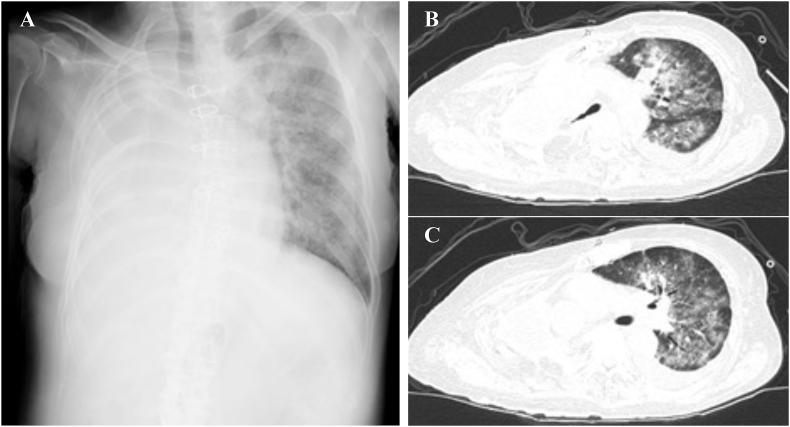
Chest X-ray and CT on urgent re-admission with complaints of high fever and severe dyspnea on day 122. (A) Chest X-ray showed the extensive ground-glass opacities in the left lung field. (B, C) Chest CT showed the diffuse ground-glass opacities in the left lung field and left pleural effusion.
